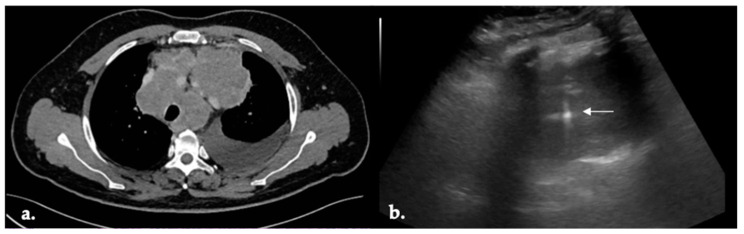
Ultrasound-guided percutaneous lung lesion biopsy (US-PLLB); Small cell lung cancer (SCLC). (a) Chest CT scan showing the target lesion; left pleural effusion was present; (b) out-of-plane 18 G US-PLLB; white arrow: needle.
